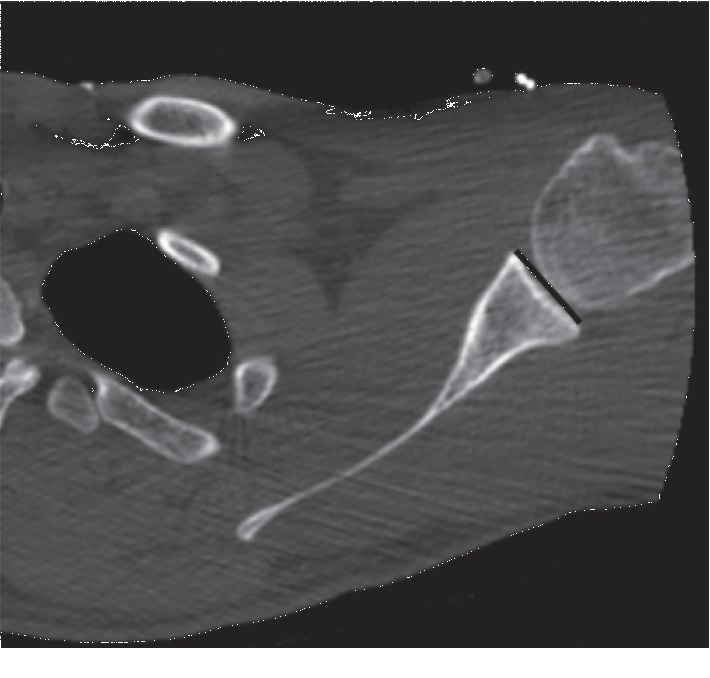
Measurement of the AP diameter (width) of the glenoid on the axial CT image.
